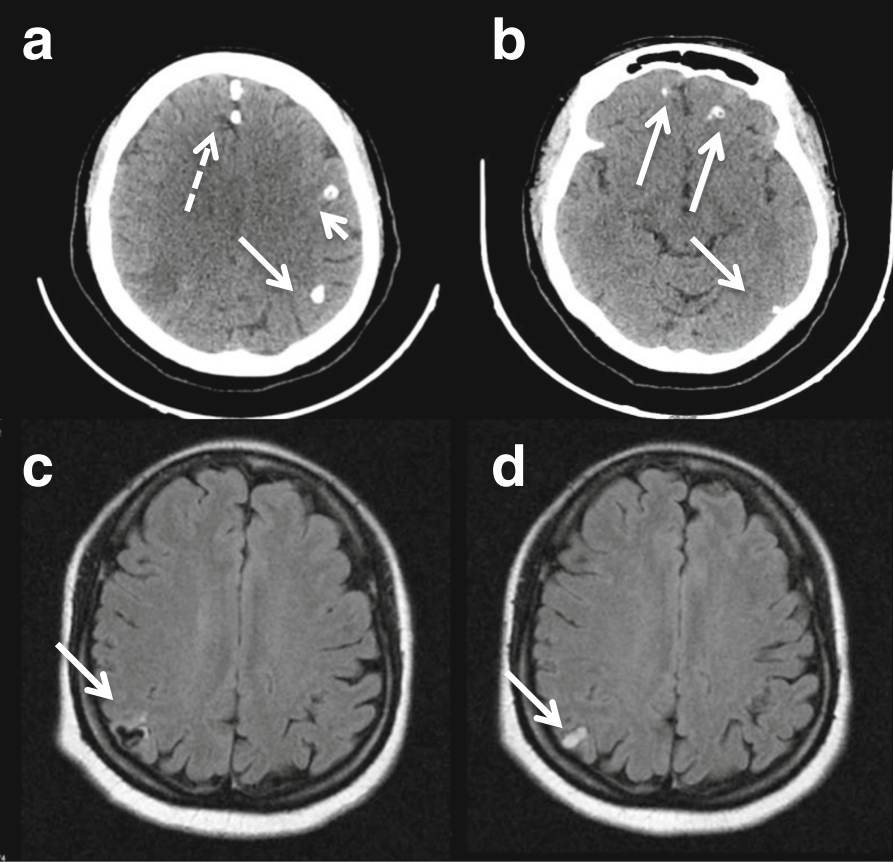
Brain CT and MRI of patient 2 before and after treatment. a and b Brain CT, multiple calcified cysts before treatment with two probably physiological falx calcifications (discontinued arrow); c MRI, FLAIR sequence, cysticercus scolex at vesicular stage before treatment; d FLAIR sequence, cyst replaced by edema after treatment
